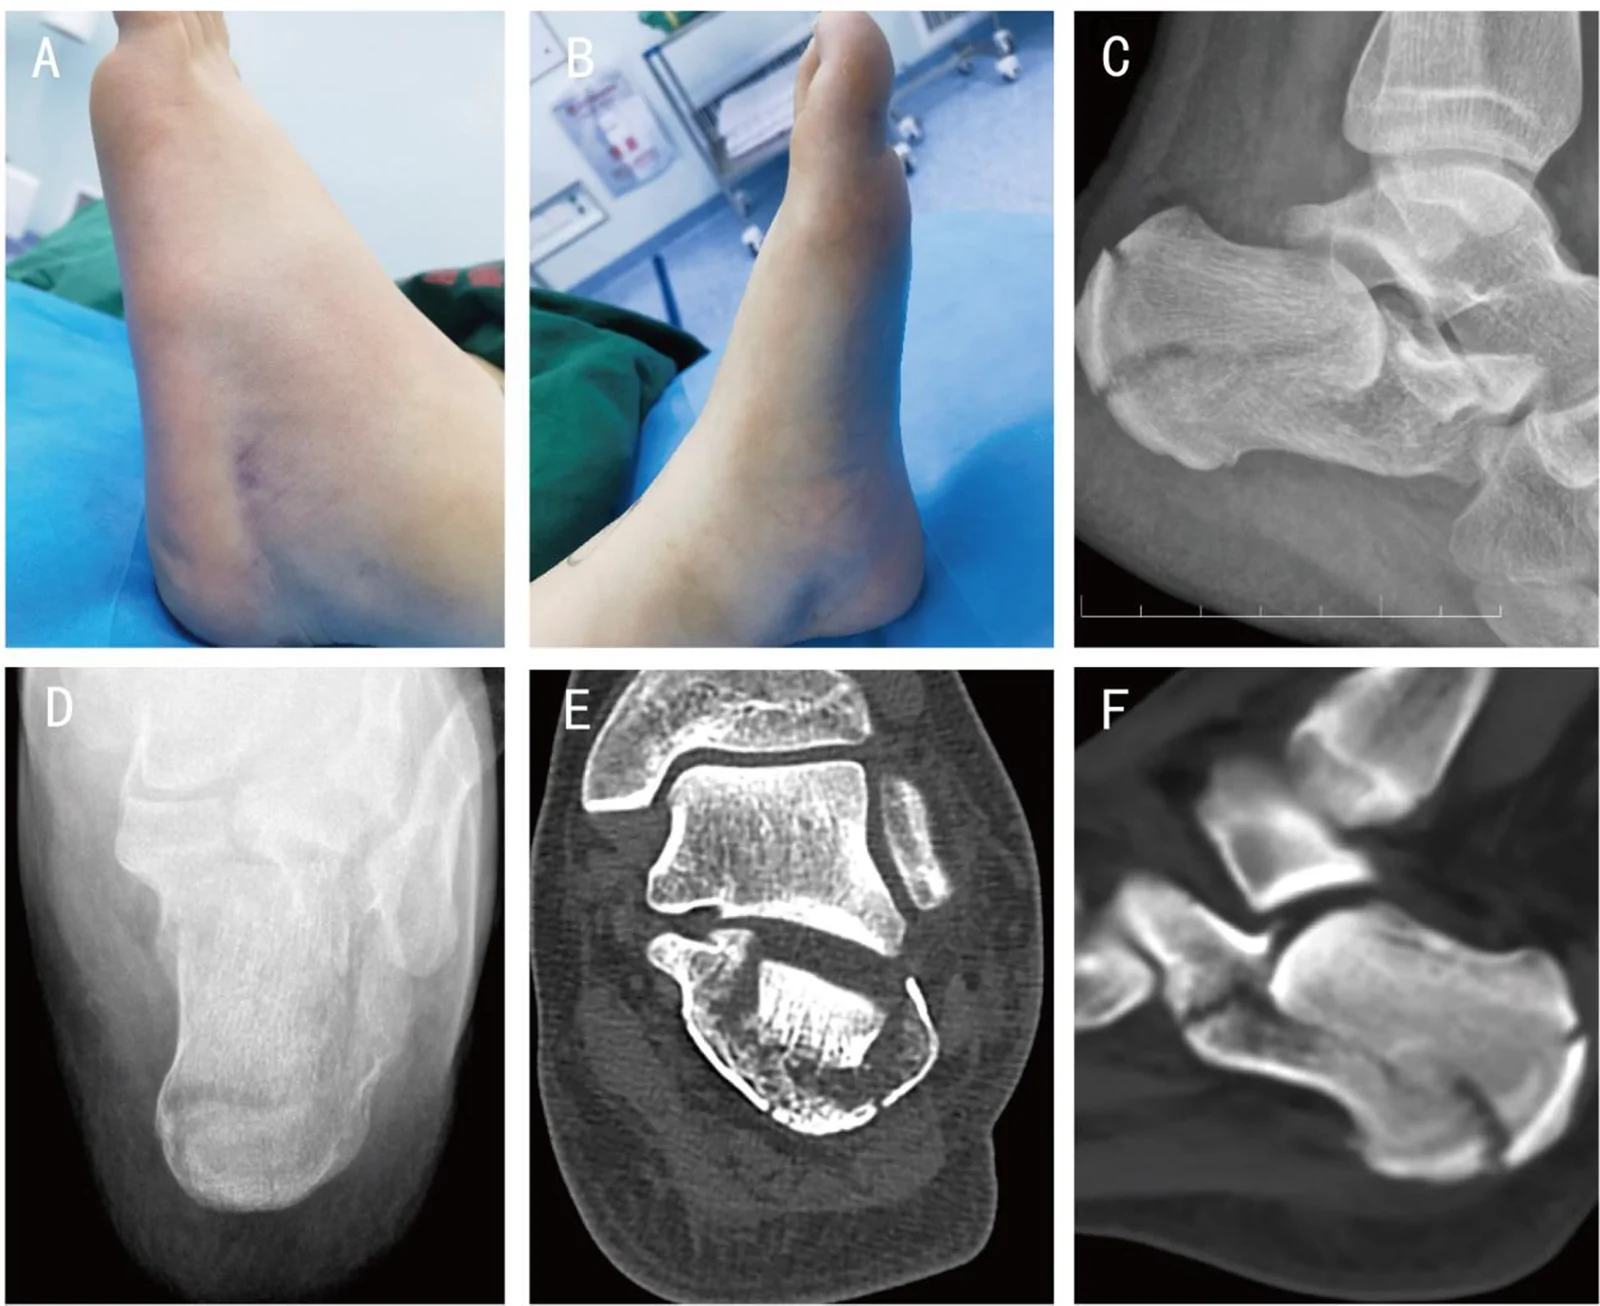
Preoperative assessment. (A) Lateral view of the left foot; (B) Medial view; (C) Lateral radiograph; (D) Axial radiograph; (E) Coronal CT; (F) Sagittal CT.
